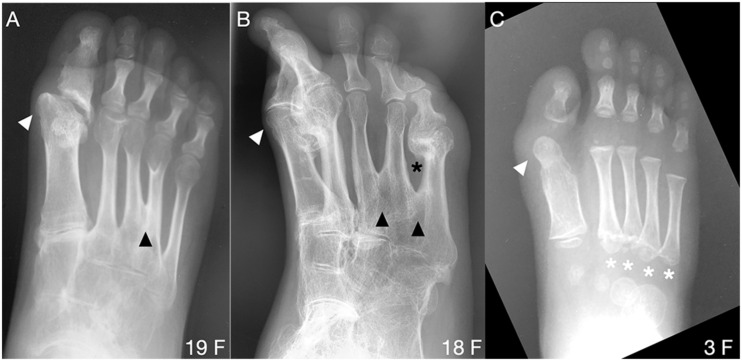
Uncommon forefoot phenotypes in FOP. (A,B) Radiographs from two patients reveal osseous syndactyly (black arrowheads) between metatarsals of digits 3 and 4 (A) and among digits 3, 4, and 5 (B). White arrowheads indicate the dysmorphic metatarsal heads (all panels), corresponding to the position of the ectopic ossification center noted in nearly all subjects with FOP. In B, extra-articular HO bridges the metatarsophalangeal joint of digit 5 (black asterisk) and HO is present in d2. (C) One of two identified patients presenting with atypical proximal metatarsal growth plates in digits 2–5 (white asterisks). Age (in years) and sex (F, M) of each subject, bottom right of each panel.
